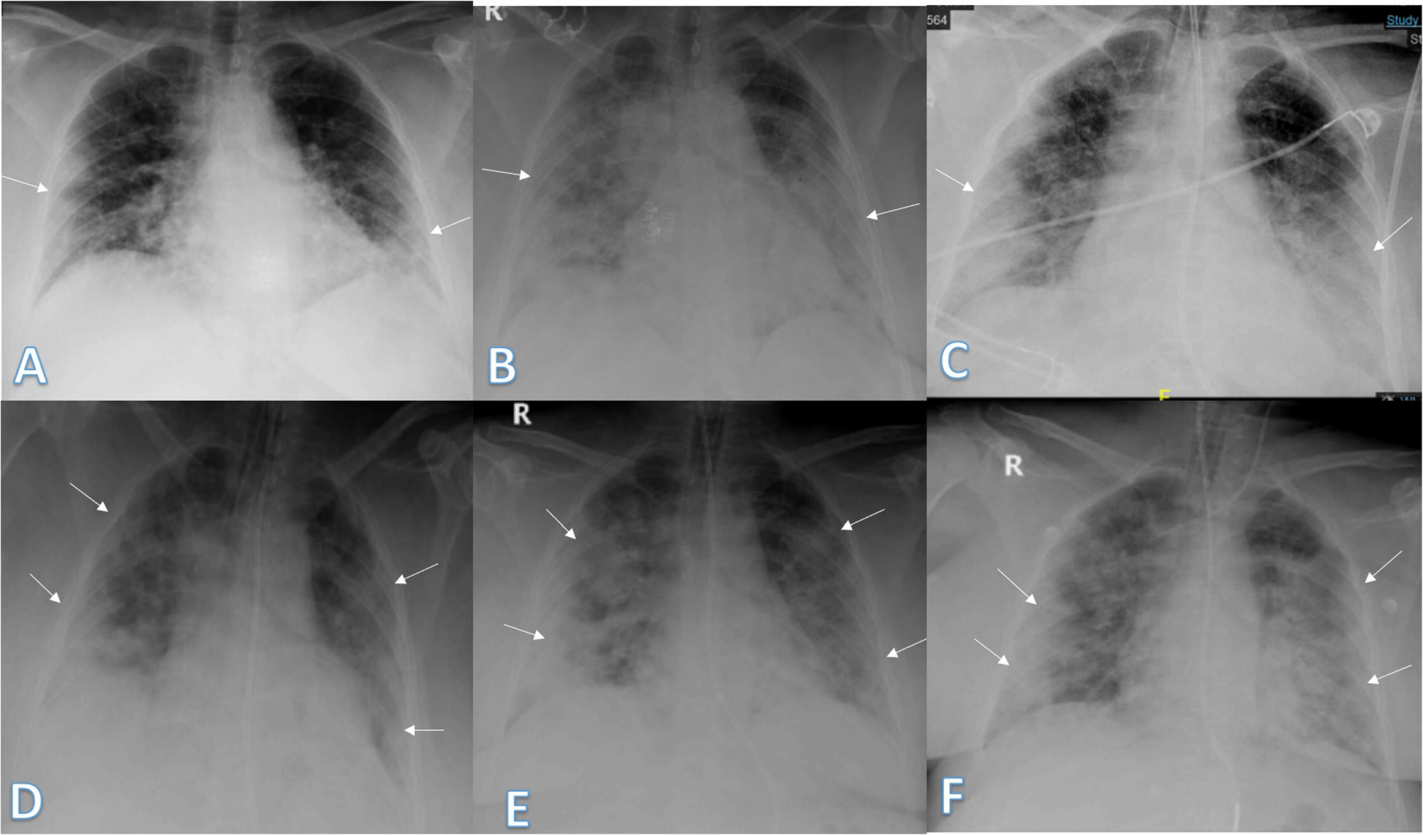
59-year female with positive COVID-19 virus. Initial X-ray (a) showed small peripheral right and left lower zonal air space consolidation opacities (arrows) with TSS as 2. On the 3rd day (b), air space consolidation opacities significantly increased extending to the upper and middle zones of both lungs (arrows) with TSS as 6 (3 on each side). The patient deteriorated and was intubated. On the 5th day (c), 7th day (d), 9th day (e), and 11th day (f), there was confluent bilateral air space consolidation opacities (arrows) with the severity score 4 on each side, so TSS was 8. The patient died on the 13th day
